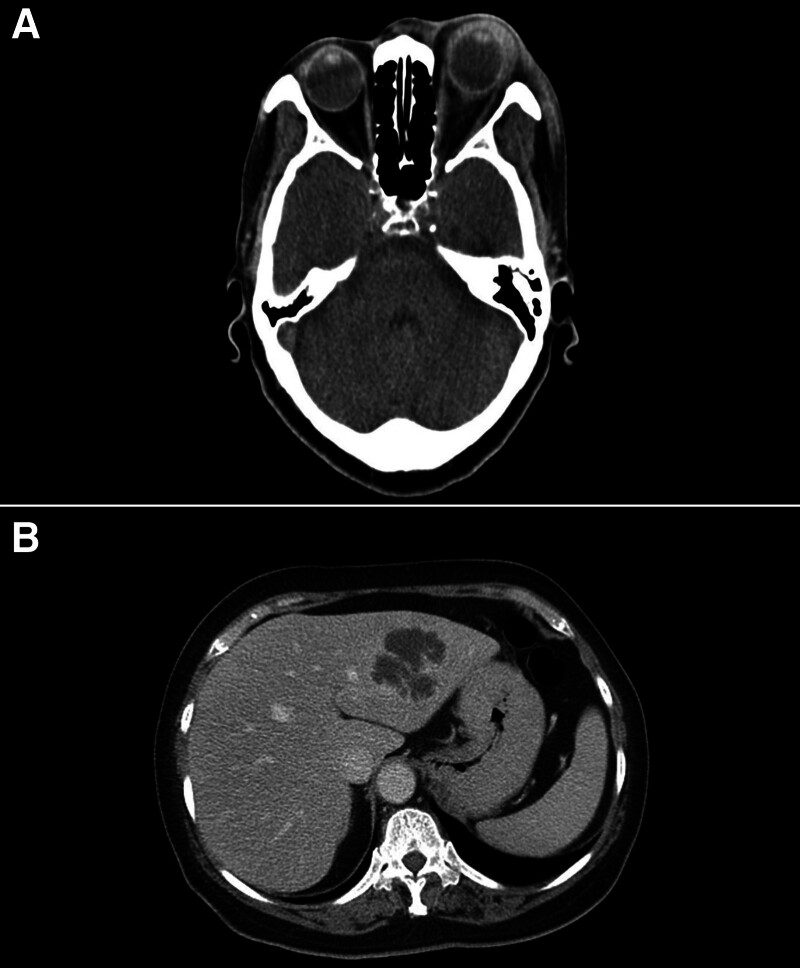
Computed tomography of the orbit (A) and liver abscess (B).
